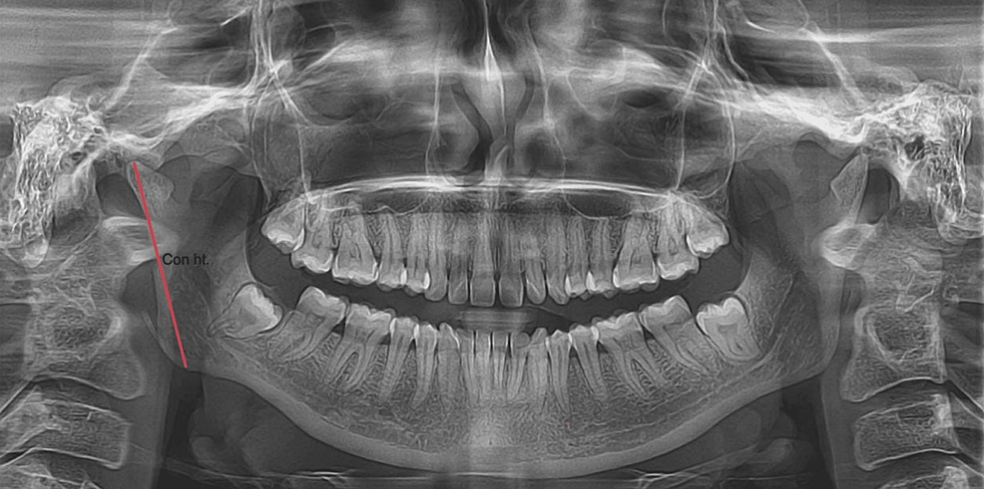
An orthopantomogram represents the condylar ramus height (Con. ht) of the mandible
